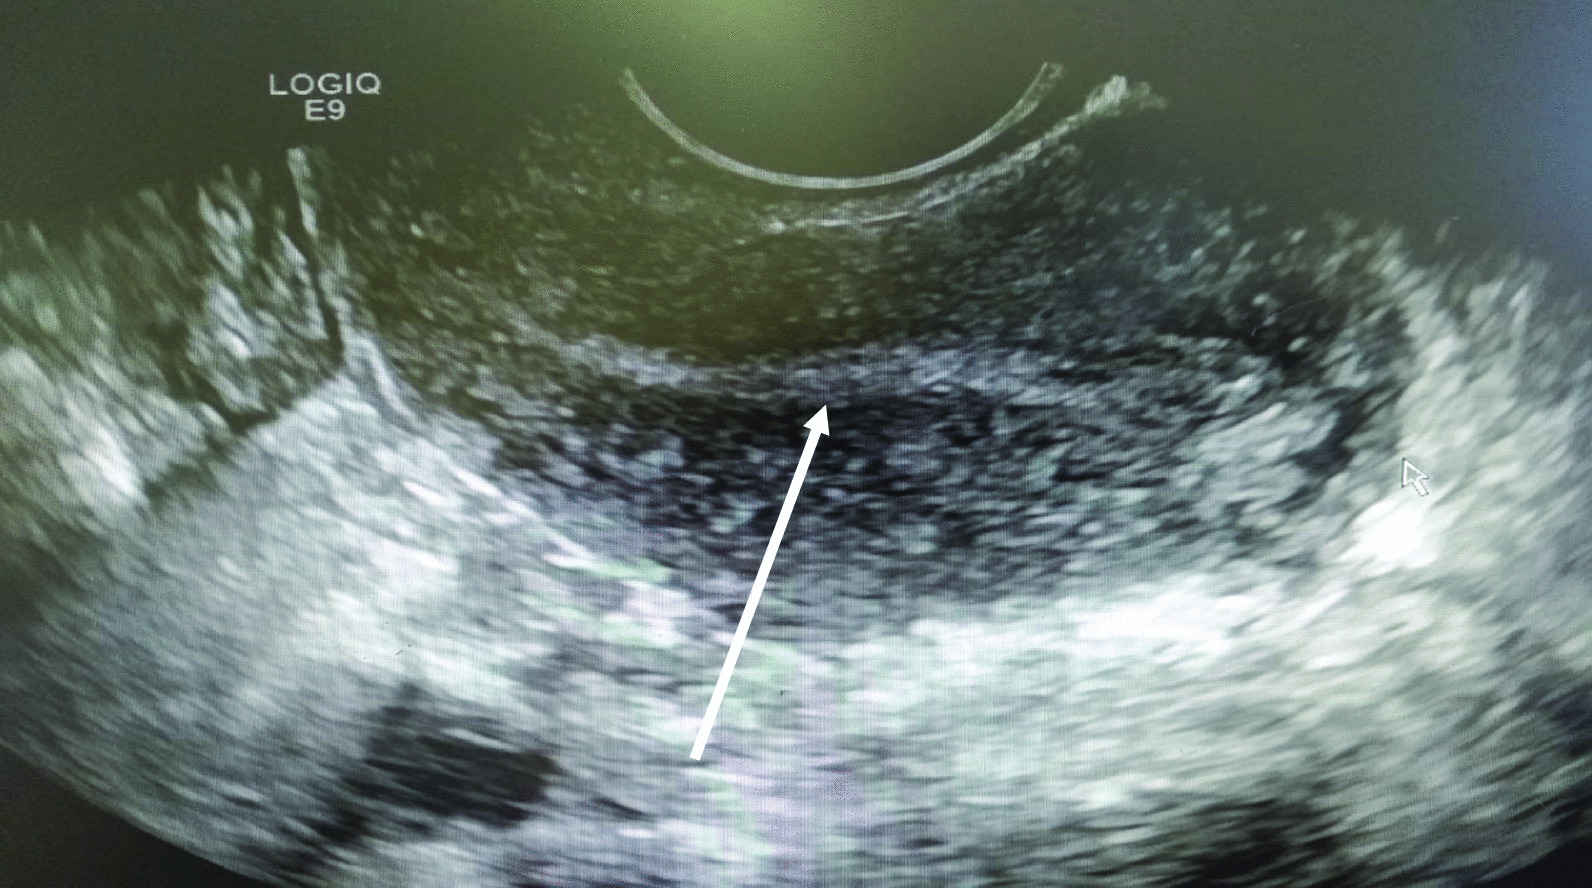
Ultrasound image of an empty uterus. The arrow points to the endometrial lining measuring 5.5 mm
